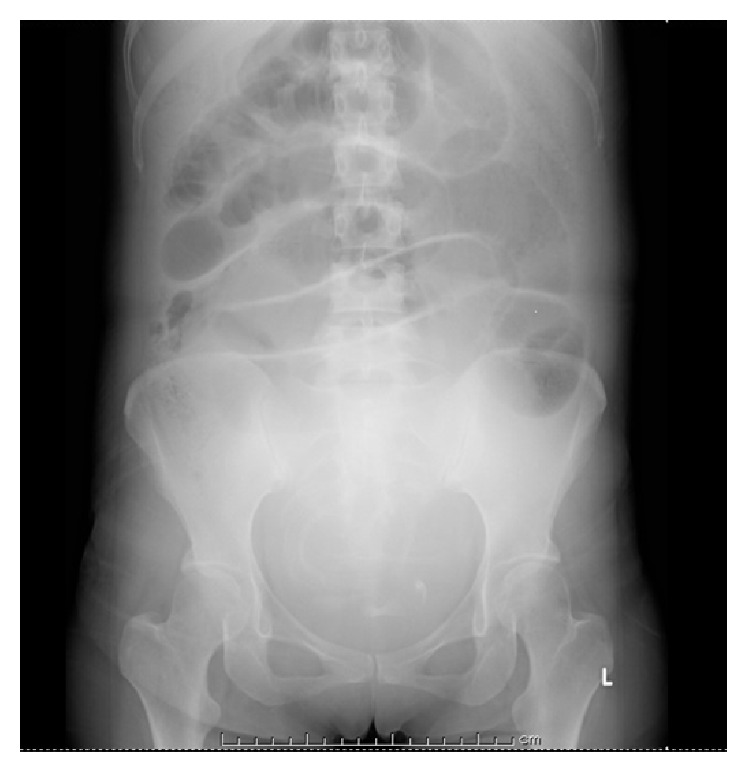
Abdominal X-ray showed air-fluid level of small intestine and dilated intestine.
